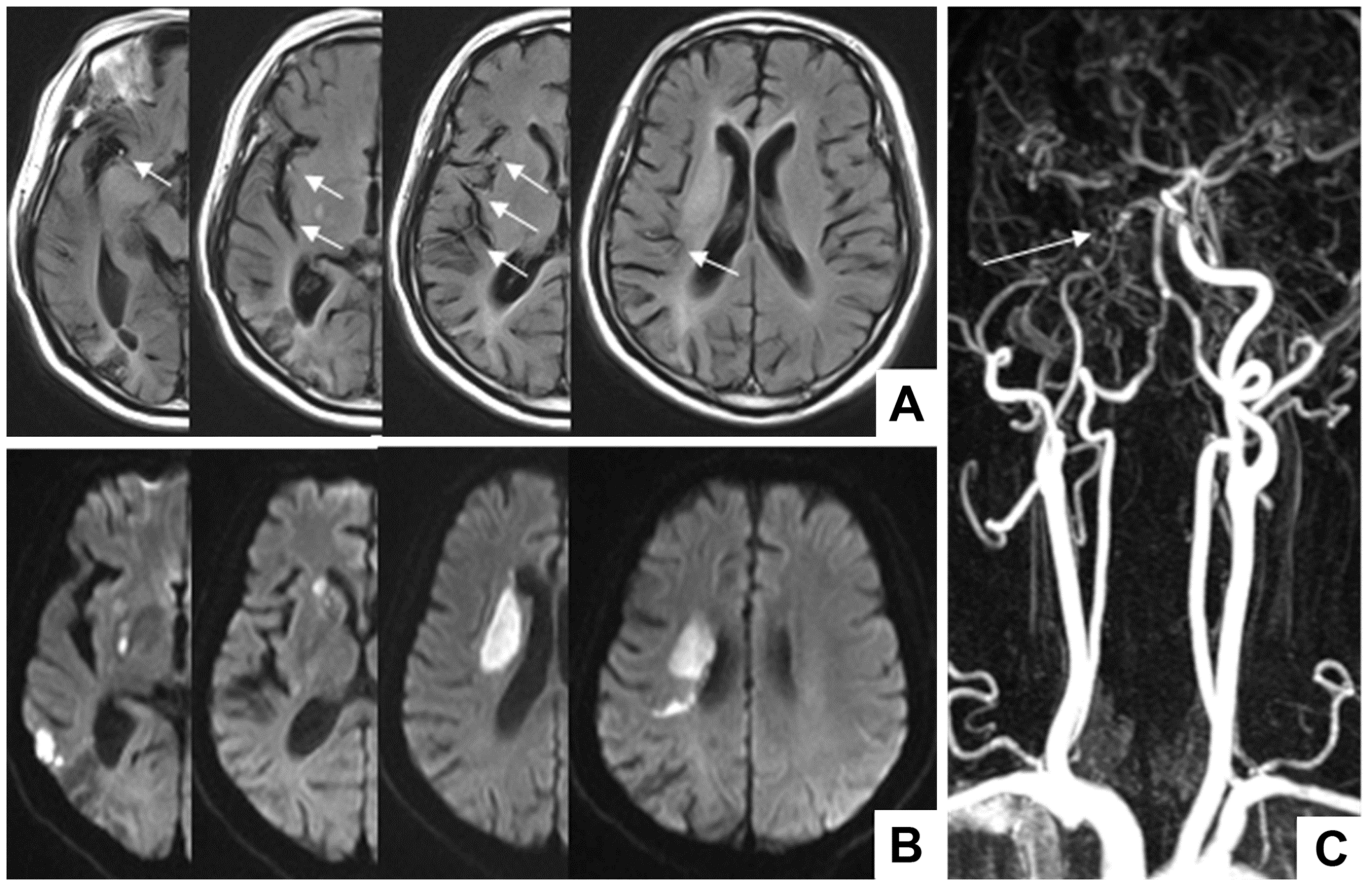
A patient with carotid artery occlusion. A: FVH was found in more than 4 images in the FLAIR sequence, which were located both at the proximal and distal segments; therefore, this patient was defined as the High FVHs and Distal FVH, respectively; B: DWI sequence indicated perforating artery infarct + cortical branch infarct; C: carotid MRA showed distal occlusion of the right internal carotid artery.
